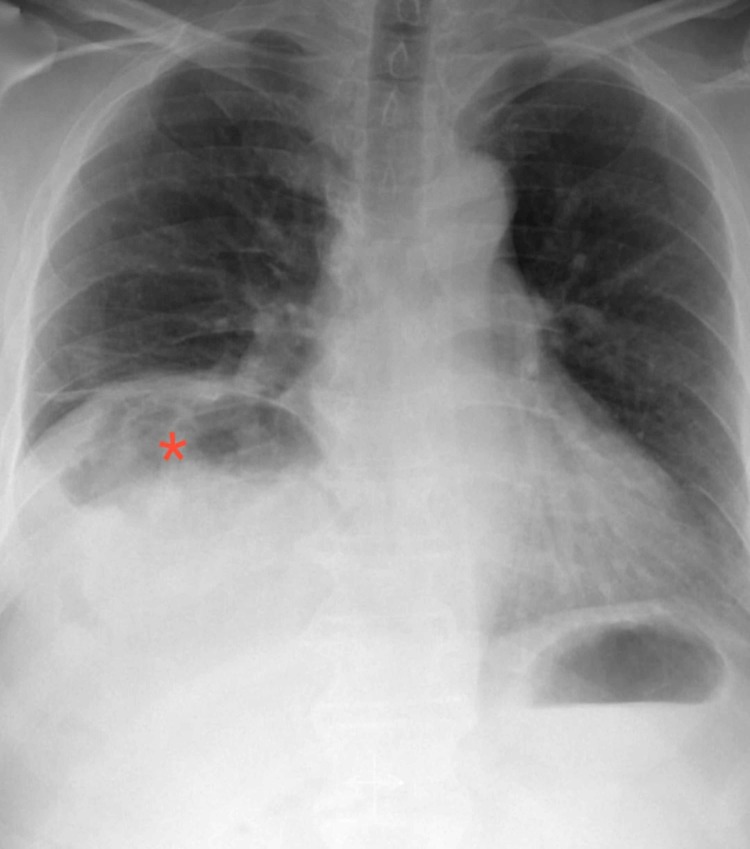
Chest X-ray showing sub-diaphragmatic gas (*) under the right hemidiaphragm
